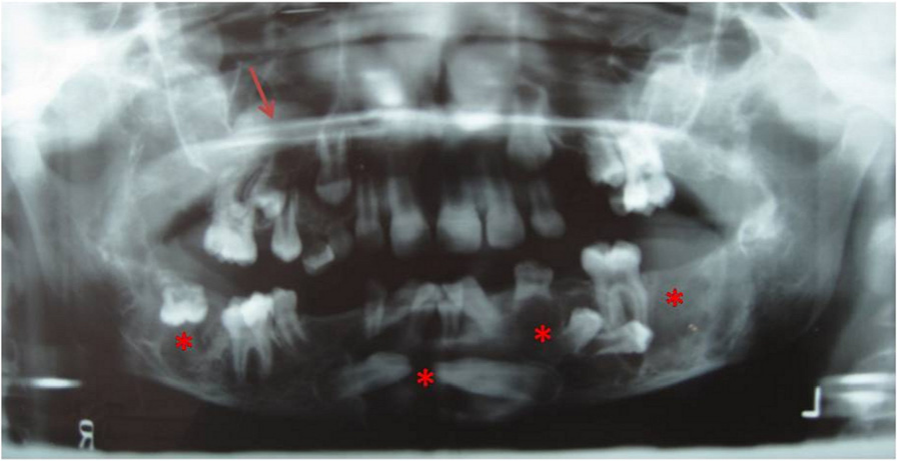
Panoramic radiography of the uncle affected.
